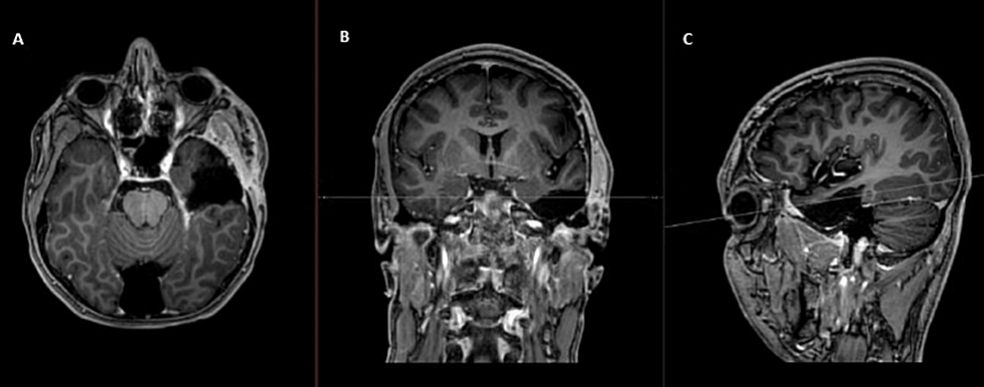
Cranial MRI one month after left temporal lobectomy (T1 sequences without contrast) (A) Axial view; (B) coronal view; (C) sagittal view Image courtesy: Al Zahraa Hospital University Medical Center (ZHUMC) Radiology Department
